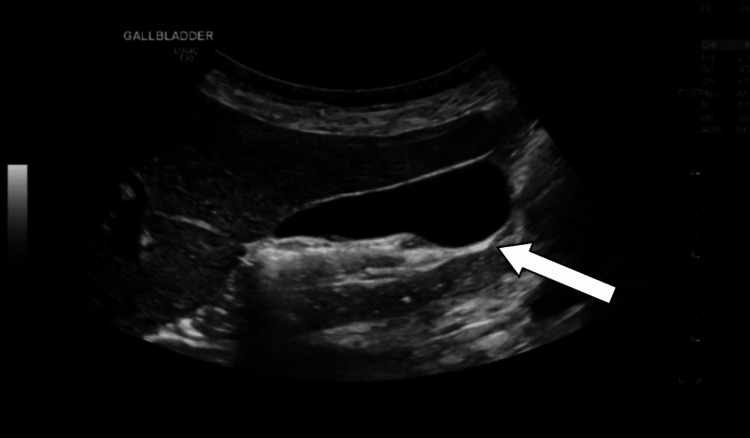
Ultrasound image obtained before colonoscopy showing the gallbladder (arrow) with normal appearance.
